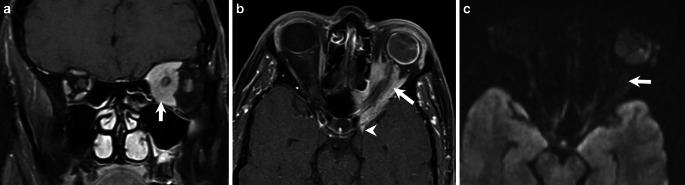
Follow-up MRI of the orbit in November 2024. Coronal T1-weighted contrast-enhanced fat-suppressed (T1 C+ FS) MR image (a) demonstrated enhancement of the left orbital mass, which appeared eccentric, suggesting possible growth beyond the confines of the optic nerve sheath. The medial portion encased the non-enhancing optic nerve (arrow). Axial T1 C+ FS image (b) showed a contrast-enhancing lesion surrounding the intraorbital and intracanalicular segments of the left optic nerve (arrow), with a dural tail visible along the sphenoid lesser wing (arrowhead). Axial diffusion-weighted images with b 1000 maps revealed no diffusion restriction of the solid tumor mass (arrow)
